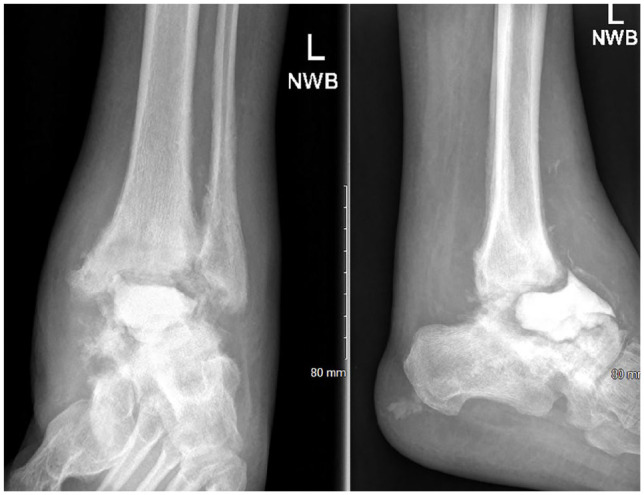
Preoperative radiographs of a patient with infected talar osteonecrosis with cement spacer in situ—bony defect measuring >4 cm.
